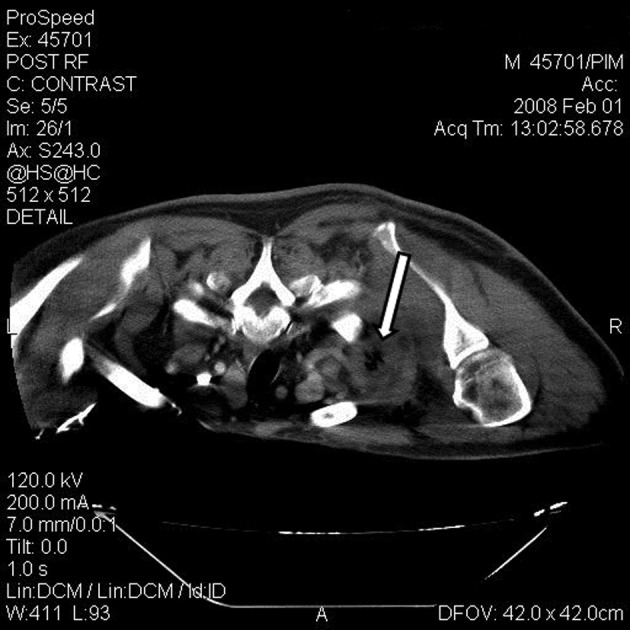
Visualization of coagulation necrosis in the centre of the mass (arrow) with a contrast-enhanced computed tomography (CT) scan immediately after the radiofrequency ablation (RFA) with the patient in prone position.
